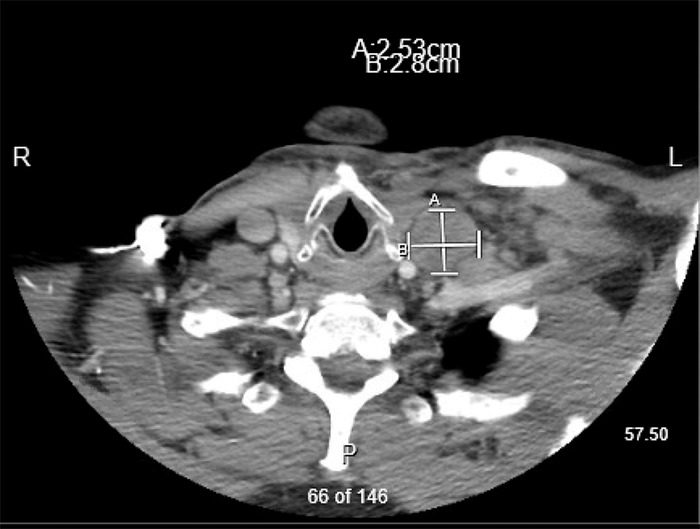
Computed tomography soft tissue neck scan revealed an enlarged left cervical lymph node measuring 2.5 × 2.8 cm and compressing the left common jugular vein.
